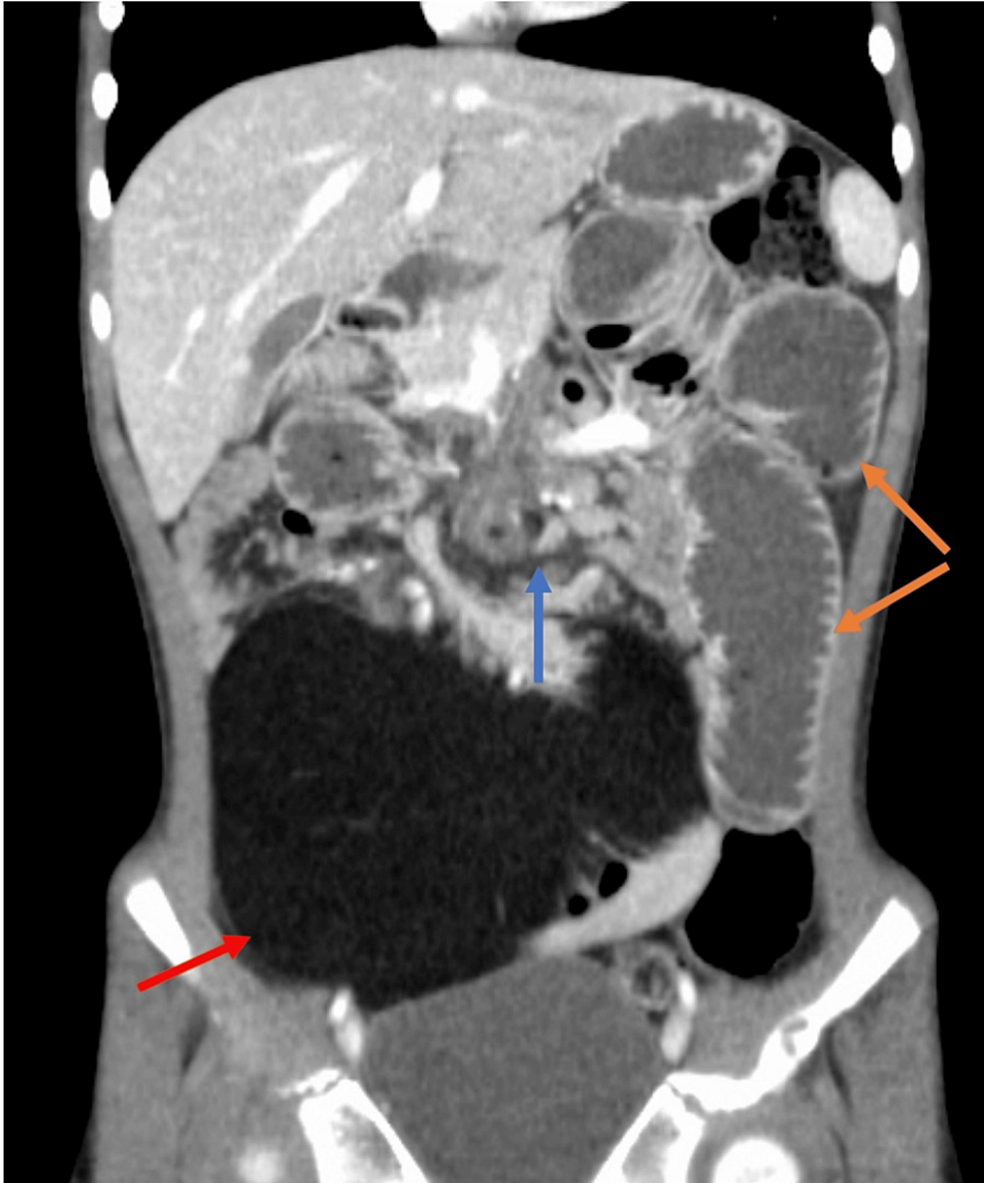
Repeat CT scan of the abdomen and pelvis Red arrow: Hypodense mass, Blue arrow: Mesenteric swirl sign, Yellow arrows: Dilated loops of small bowel
